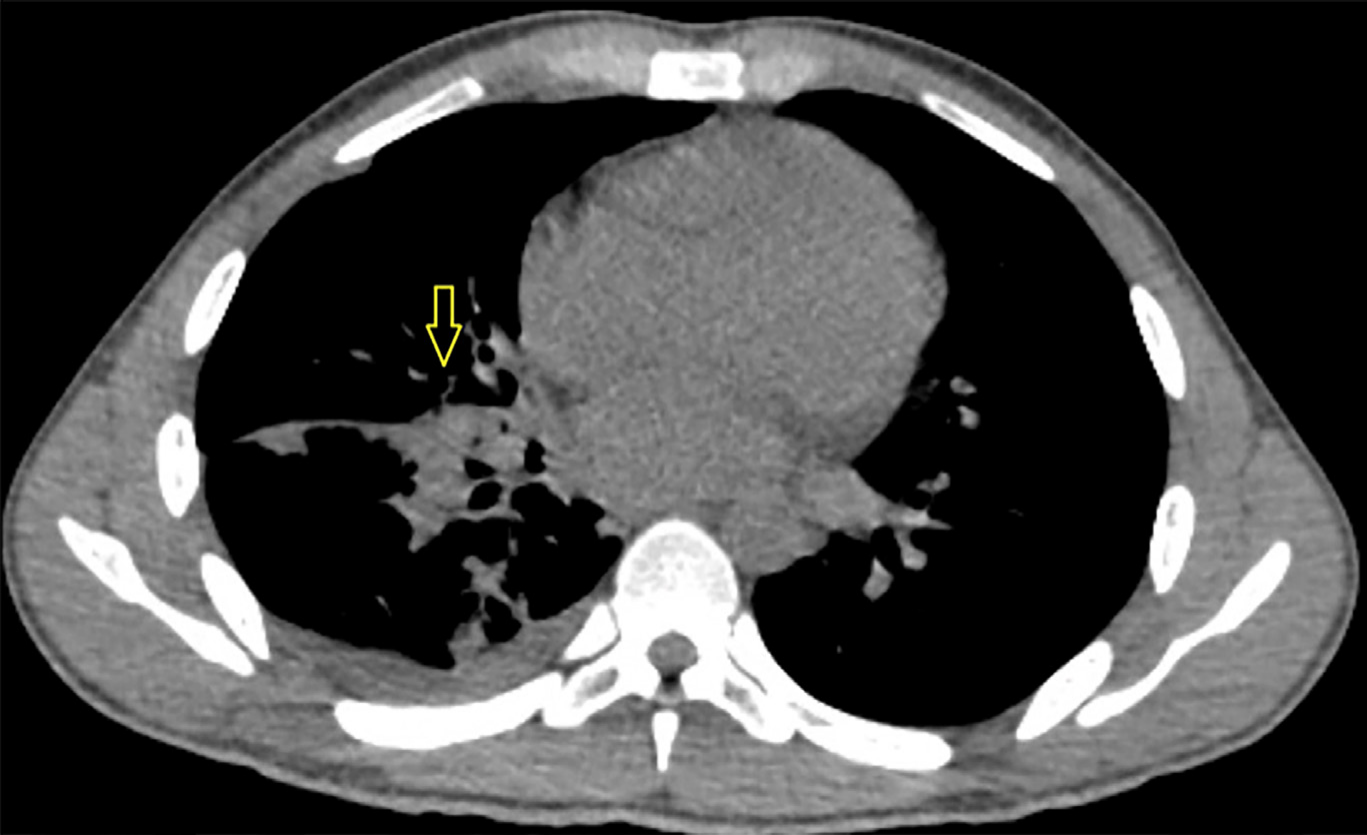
Repeat CT chest without contrast demonstrating decrease in size of right perihilar mass.
